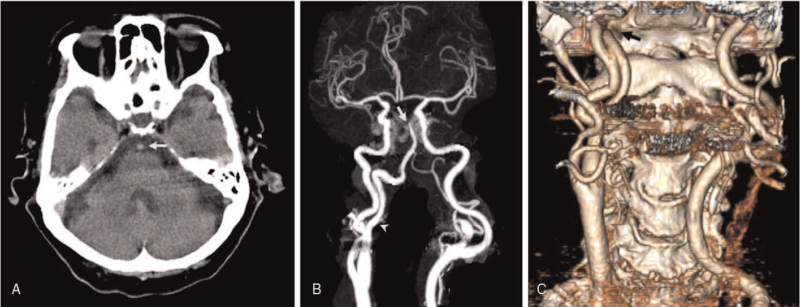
(A) Non-enhanced head computed tomography (CT) reveals a hyperdense basilar artery (white arrow). (B) CT angiography (CTA) reveals complete occlusion of the distal basilar artery (white arrow) and dilated branch arising from the right internal carotid artery (white arrowhead). (C) Three-dimensional reconstruction imaging of CTA shows a dilated branch arising at the C2 level, extending posteriorly and passing through the enlarged hypoglossal canal (black arrow).
